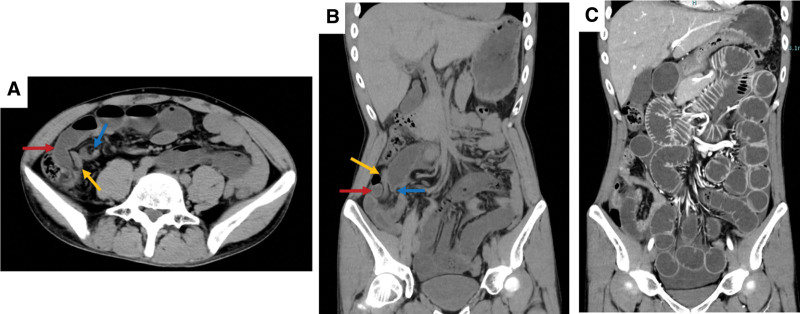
Preoperative computed tomographic (CT) findings are presented. (A and B) Non-contrast CT reveals a narrowing of the terminal ileum (blue arrow), with dilatation of the proximal small bowel. Dilated bowel with a blind end (red arrow) and band-like structure surrounding the small bowel constriction, with a collapse of the distal small bowel (yellow arrow), are shown. These findings are suggestive of an internal hernia, due to a mesodiverticular band of the Meckel’s diverticulum. Signs of strangulation are not evident. (C) Contrast-enhanced CT reveals no evidence of closed-loop obstruction or intestinal ischemia. The dilated bowel loop suggestive of a Meckel’s diverticulum that has been observed on non-contrast CT cannot be confirmed by contrast-enhanced CT.
